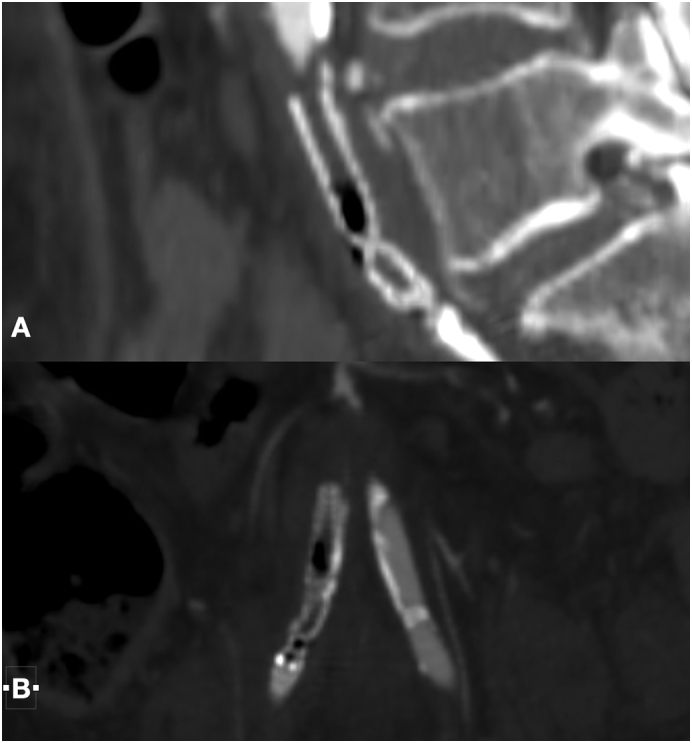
CT angiography MPR with detail of gas bubbles inside the stent-graft in common iliac arteries through the sagittal plane (A) and coronal plane (B).
